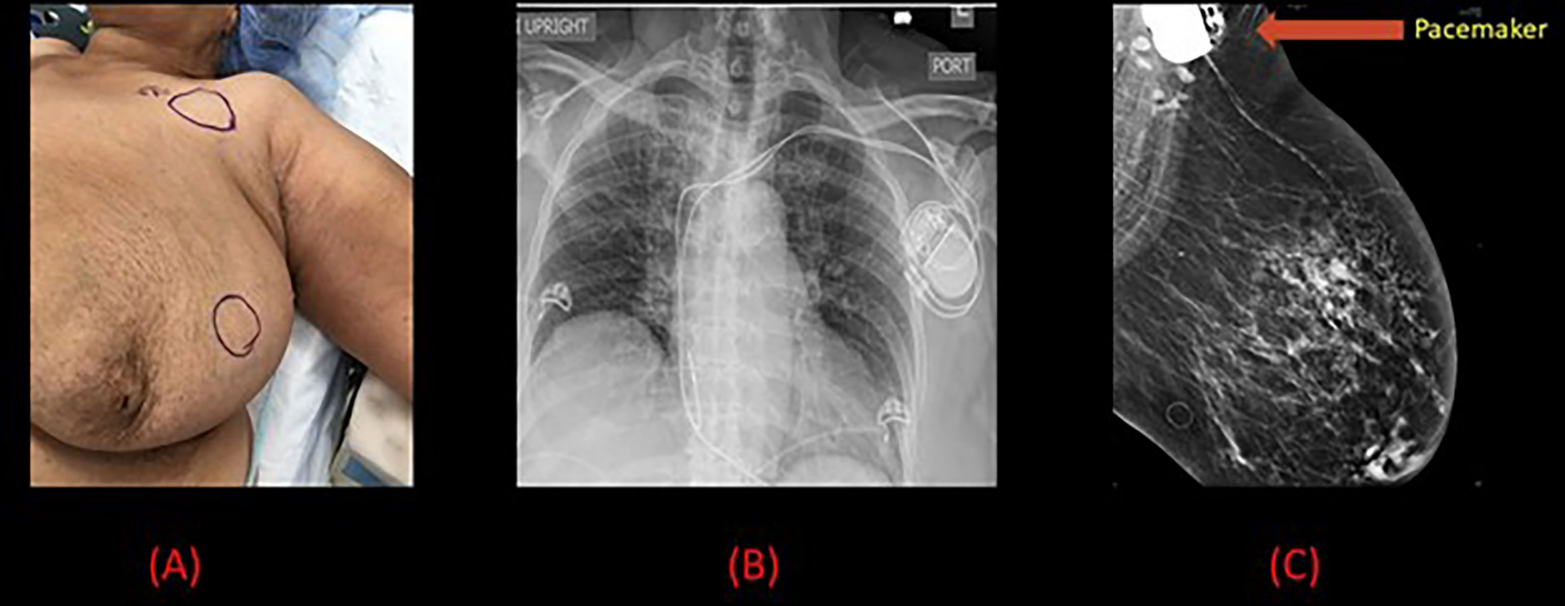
Pre-operative and radiological images for patient 1. (A) Pre-operative image of the left breast with the location of the pacemaker marked by a circle in the superior portion close to the neck and the inferior circle indicates the presence of radiological seed. (B) Chest x-ray demonstrating left sided pacemaker with dual leads projecting over the right atrium and right ventricle. (C) Mammogram demonstrating a 10 mm spiculated mass in the left breast along with the pacemaker.
